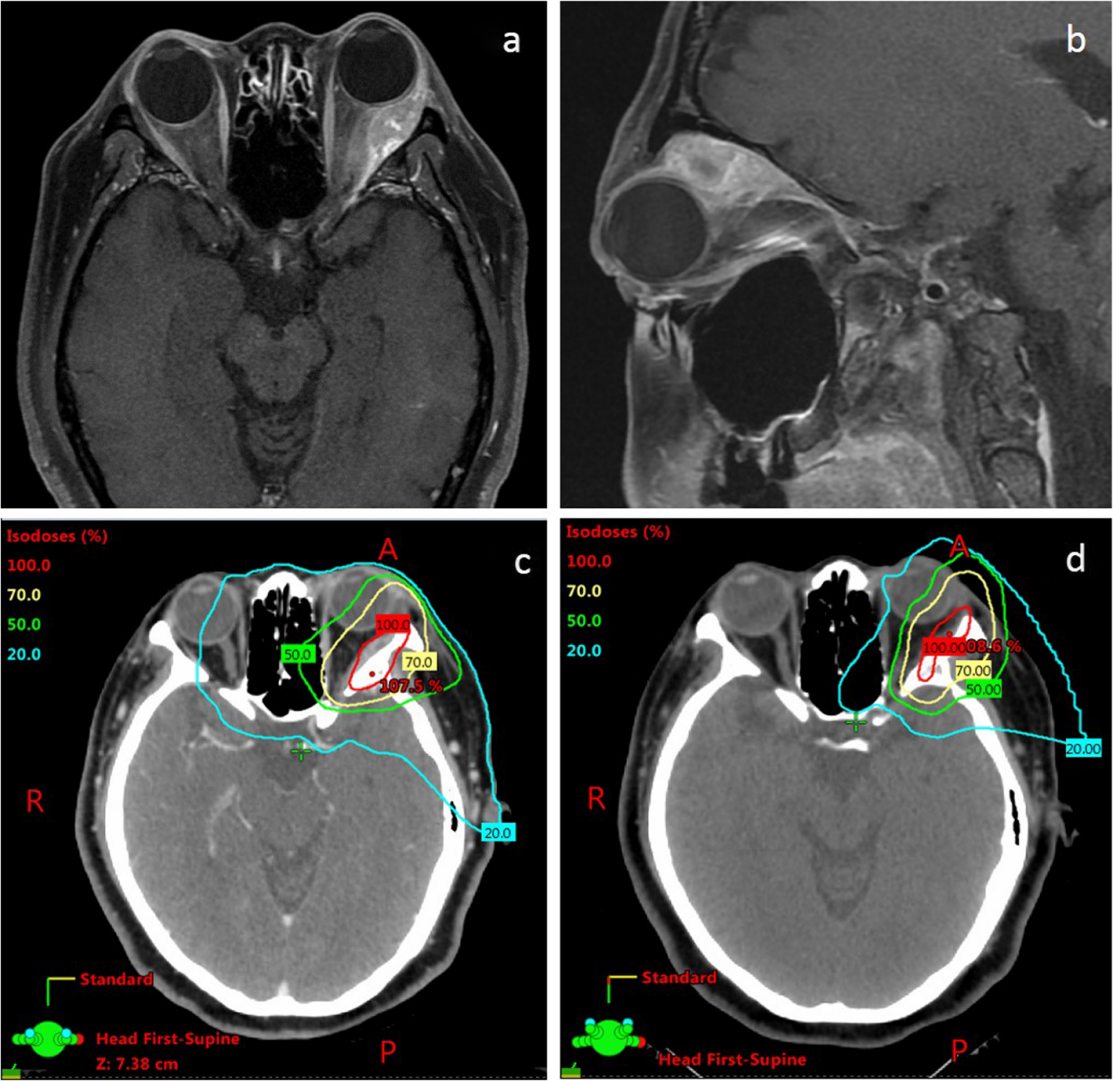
Patient number 7, cT2bN0M0 lacrimal gland cancer, pre-surgery T1-MRI and the photon or proton beam dosimetry of adjuvant radiotherapy. Total RT dose was 70 Gy in 33 fractions. Dose constraints for organs at risk: Brain stem: D_max_ < 54 Gy. Left eye and left optic nerve: D_max_ ≤ 59.5Gy (85% dose). Right eye and right optic nerve: D_max_ ≤ 50 Gy. Optic chiasm: D_max_ < 54 Gy. Right len: D_max_ ≤ 18Gy. No ocular complication was noted during our study period. a. Axial view of the tumor. b. Sagittal view of the tumor. c. Photon beam dosimetry. 1d. Proton beam dosimetry
